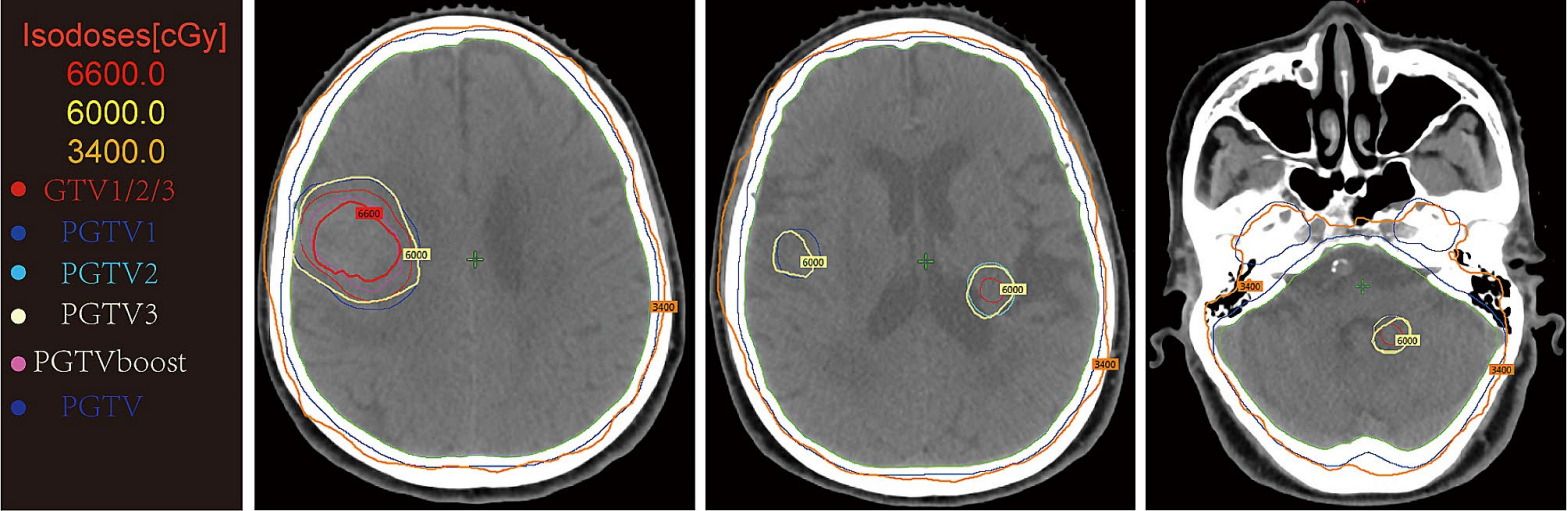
The location of brain tumors from positioning CT images and final excellent dose distribution for each tumor
